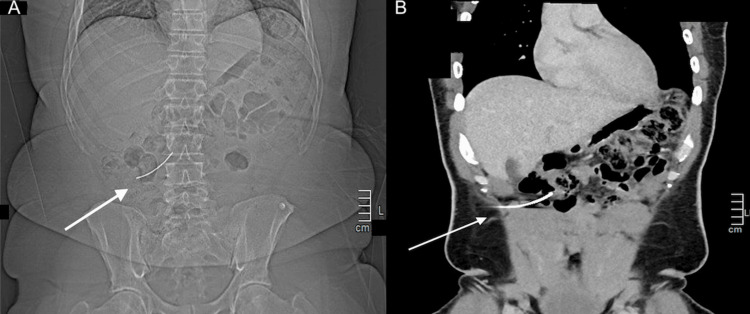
(A) Scout film before CT with the arrow pointing to the foreign body. (B) CT image with the arrow pointing to the foreign body.
